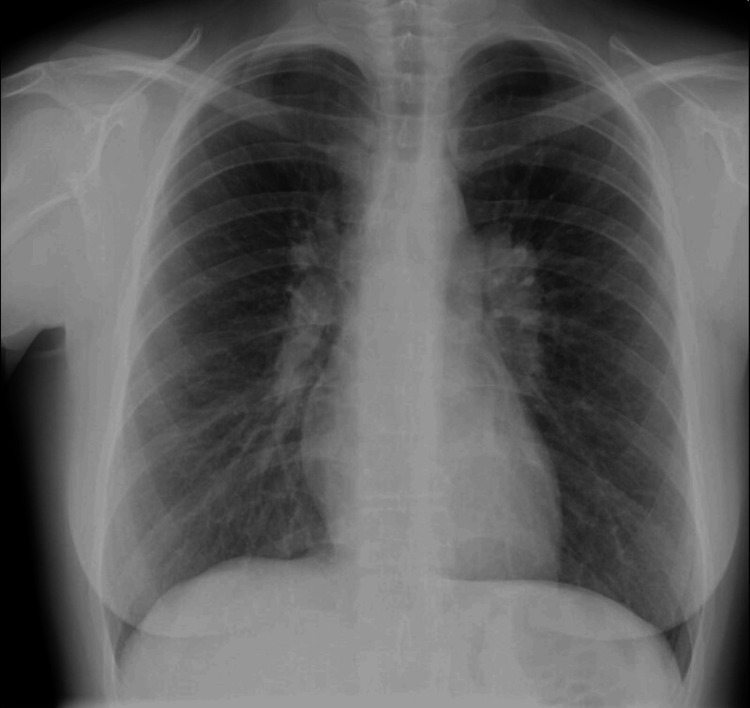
Thorax X-ray showing bilateral hilar lymphadenopathies
